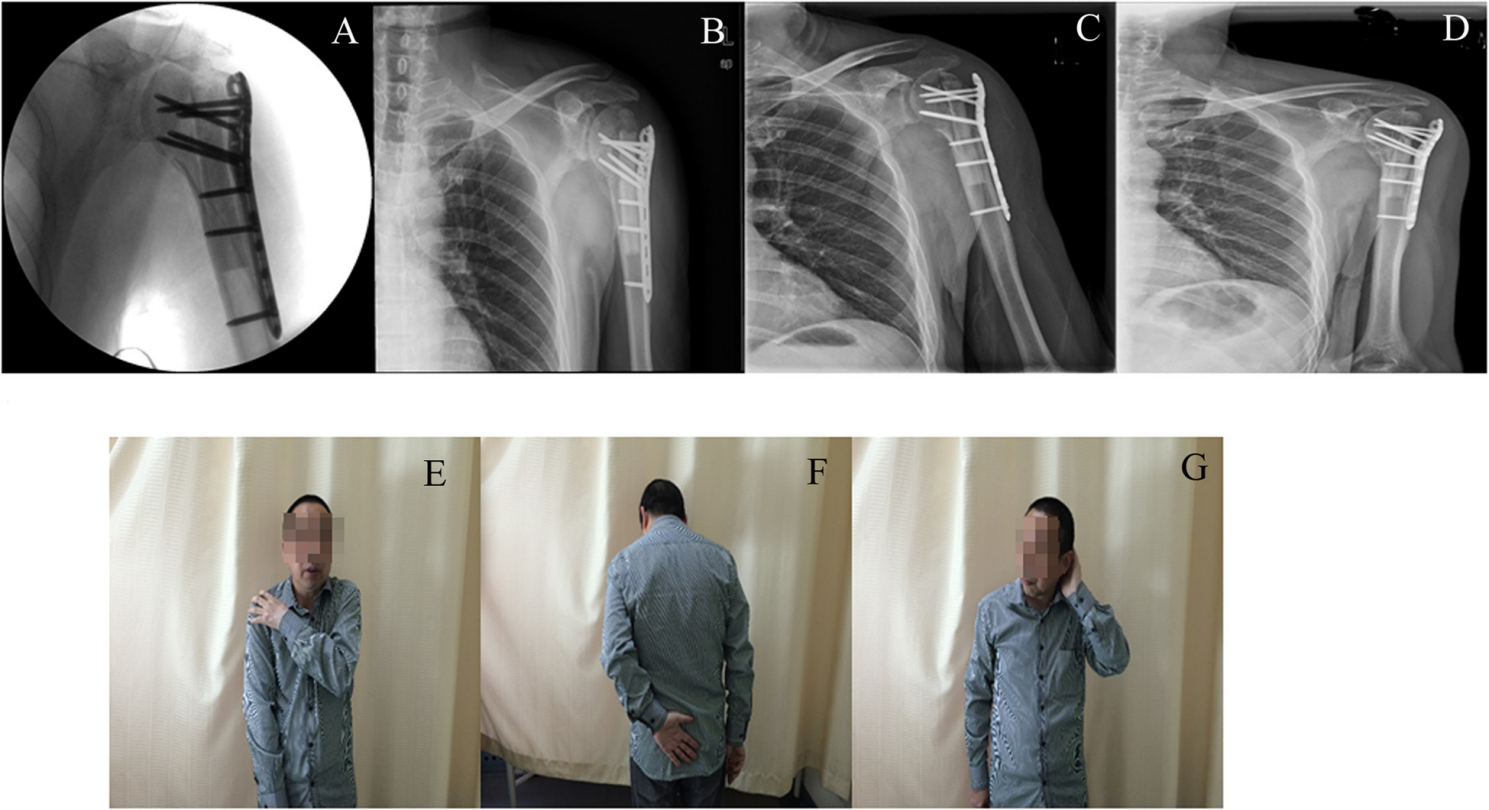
One case develops varus displacement but acquires good function without need of revision. a X-ray film before surgery. b X-ray film 3 days after surgery. c X-ray film 3 months after surgery. d X-ray film 38 months after surgery. e-g Function of patient’s upper arm
